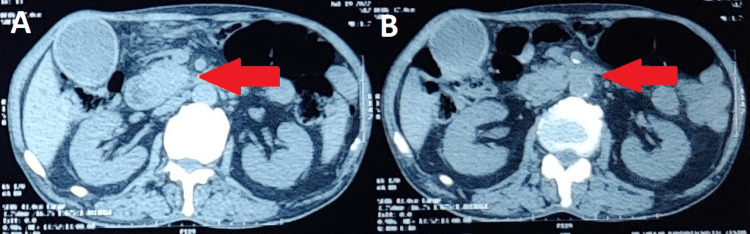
Different views (A and B) of CT scan showing a soft tissue density lesion in the head of the pancreas
